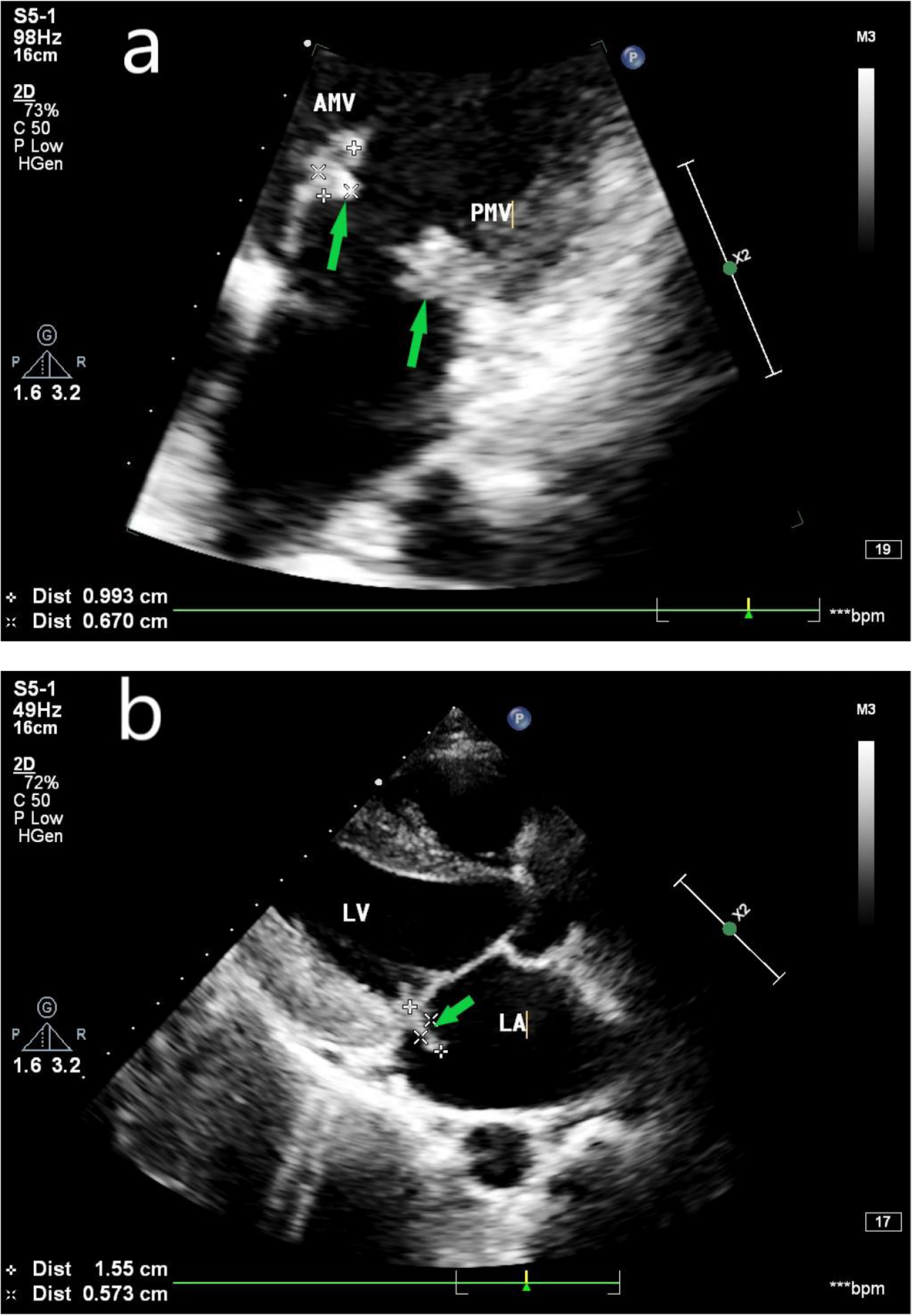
a Echocardiography at 26 weeks of gestation shows that the anterior leaflet of the mitral valve is thickened, is rough, and has vegetative formation. b. The posterior leaflet shows vegetative formation (AMV = anterior leaflet of the mitral valve; PMV = posterior leaflet of the mitral valve; LA = left atrium; LV = left ventricle; green arrow = infective endocarditis vegetative)
